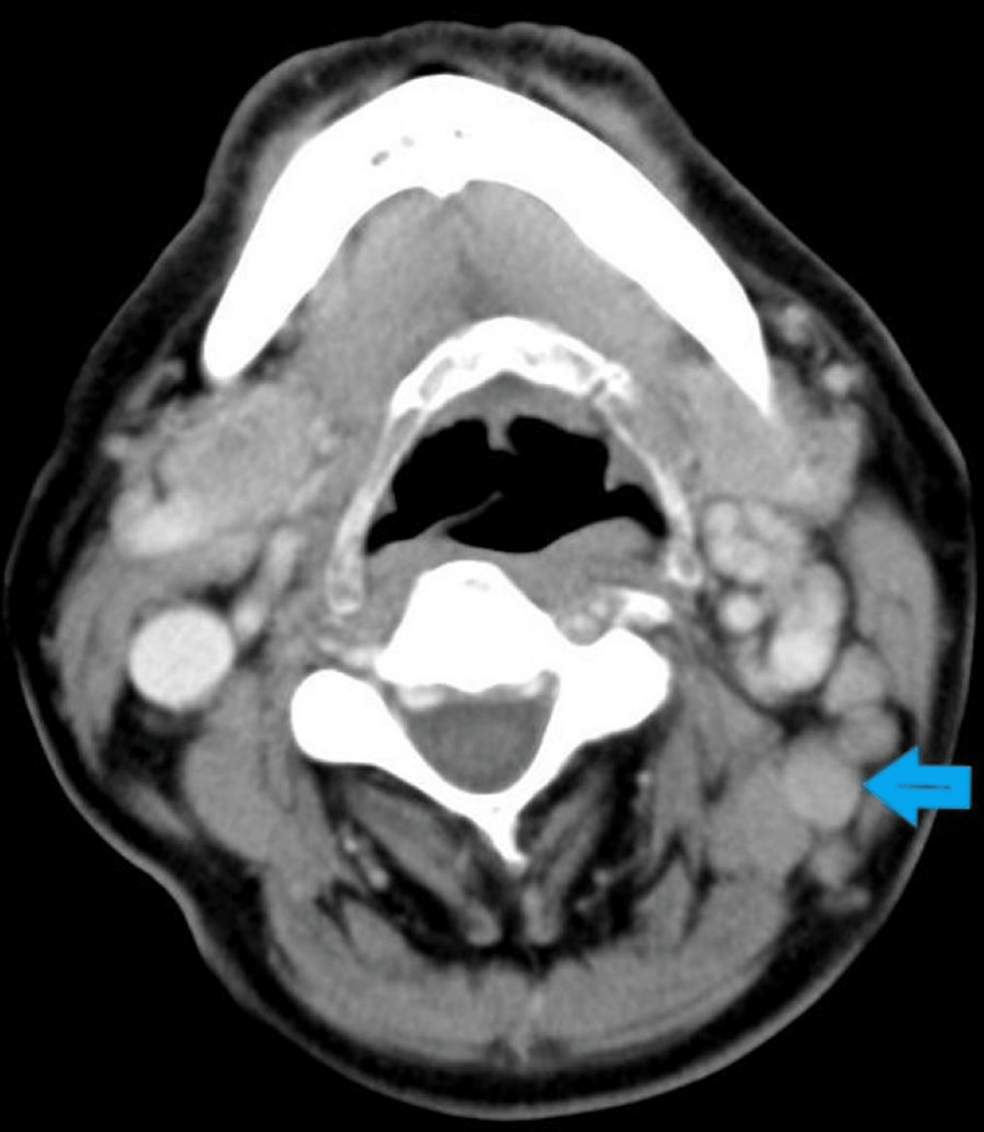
A CECT scan of the neck shows enlarged multiple left jugular lymph nodes. CECT: Contrast-enhanced computed tomography.
